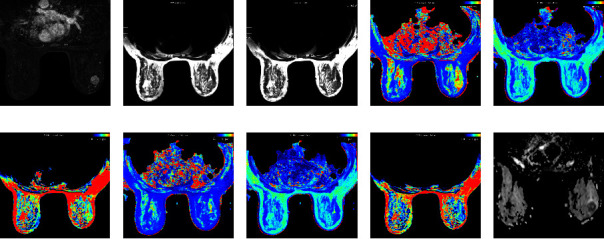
51-year-old female with invasive ductal carcinoma (IDC) of the right breast. Ki-67 expression was 50%. Axial contrast enhanced T1-weighted image (T1WI) showed an irregular heterogeneous enhancing mass with irregular margin (a) The quantitative parameters were measured on SyMRI-T2WI before ((b) pre-T1 = 1509 ms, pre-T2 = 80 ms, and pre-PD = 82 pu) and after ((c) post-T1 = 703 ms, post-T2 = 72 ms, and post-PD = 72.1 pu) administration of the contrast medium. Similar images are shown for the mass on precontrast ((d) T1 mapping, (e) T2 mapping, and (f) PD mapping) and postcontrast ((g) T1 mapping (h) T2 mapping, and (i) PD mapping) enhanced mapping images. The apparent diffusion coefficient (ADC) value was 0.763 × 10^−3^ mm^2^/s on the ADC map image (j).
